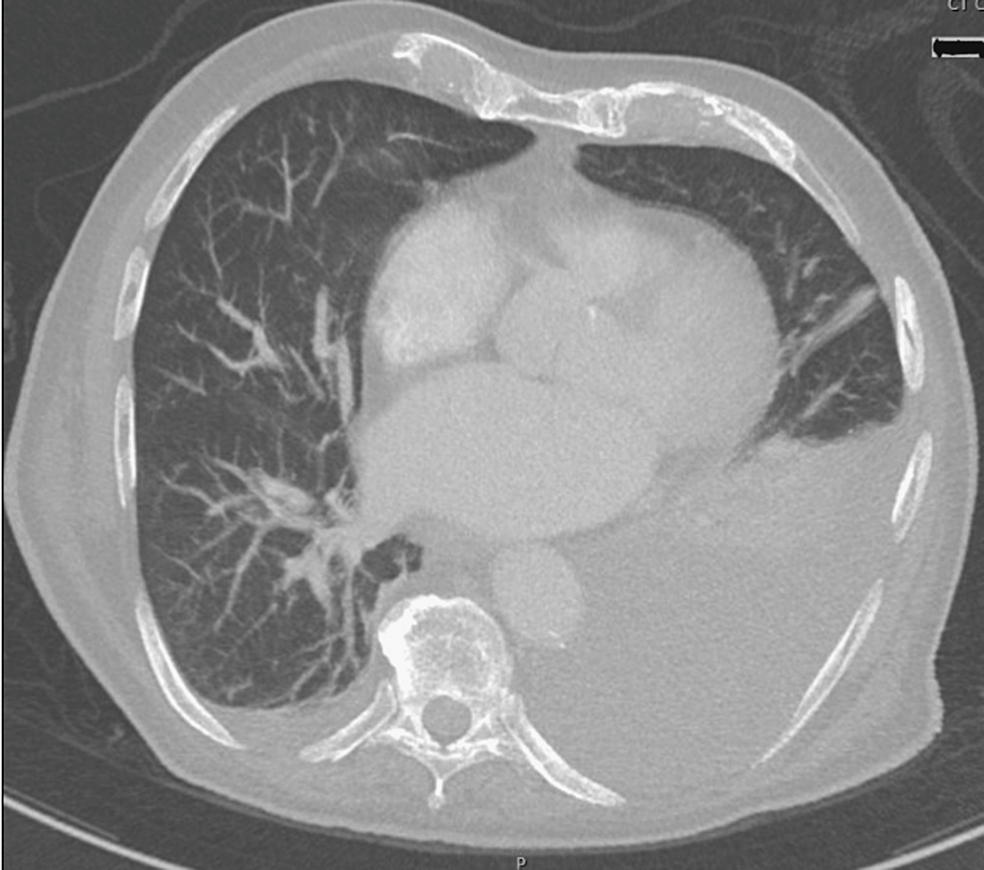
CT of the chest without contrast showing a left pleural effusion CT: computed tomography
